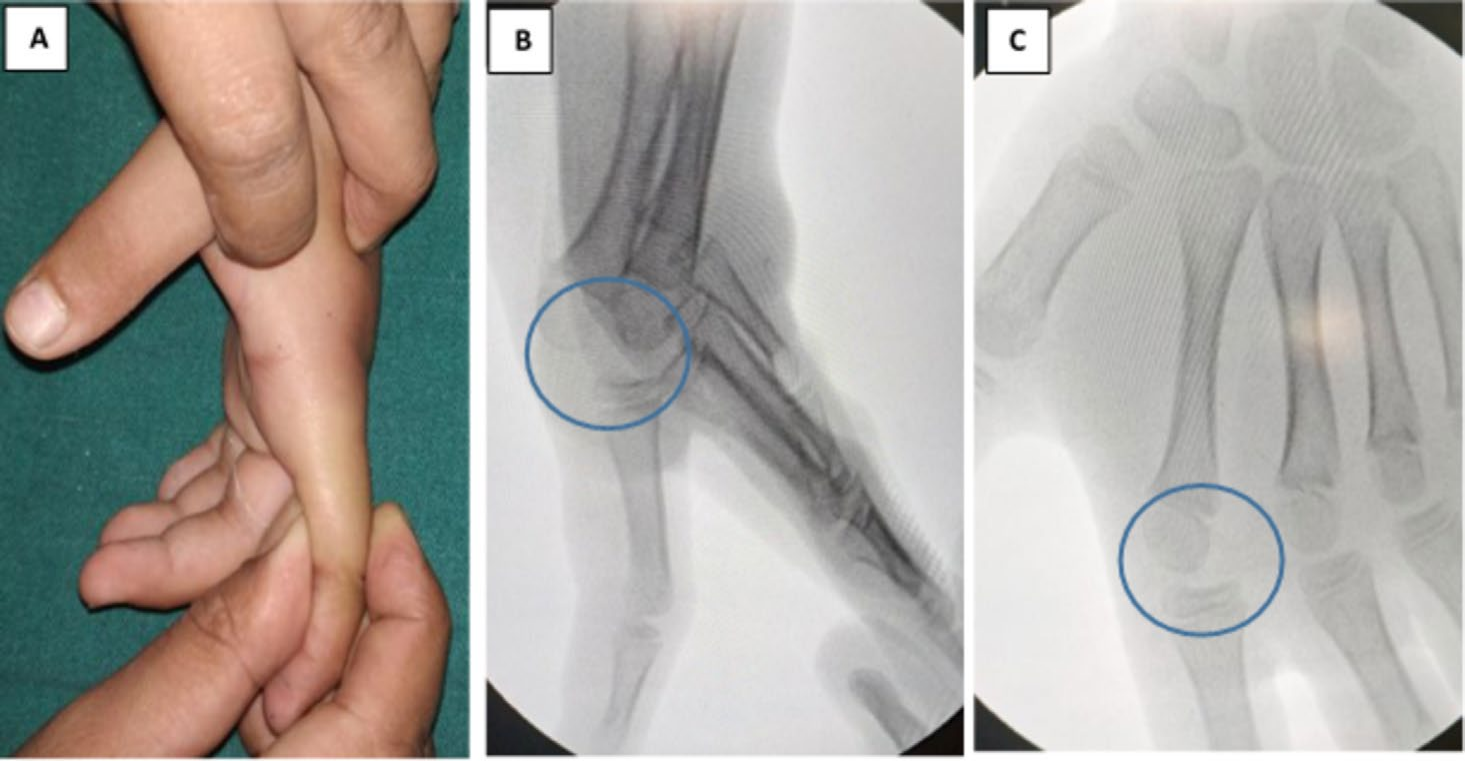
Pre‐operative clinical image (A) and radiographs (B, C) showing left hand index finger MCP joint dislocation (rings).
